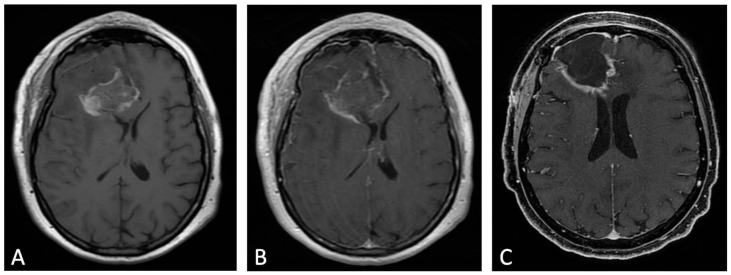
Example of evolution of thick linear CE. Pre-contrast (A) and post-contrast (B) T1w images of early post-operative MRI after surgical resection of GB; post-contrast T1w of MRI performed one month after surgery (C). Thick peripheral enhancement may be seen particularly along the medial and posterior margin of the surgical site, with obvious tumor progression consistent with an area of thick linear-nodular enhancements at the resection bed one month after surgery.
